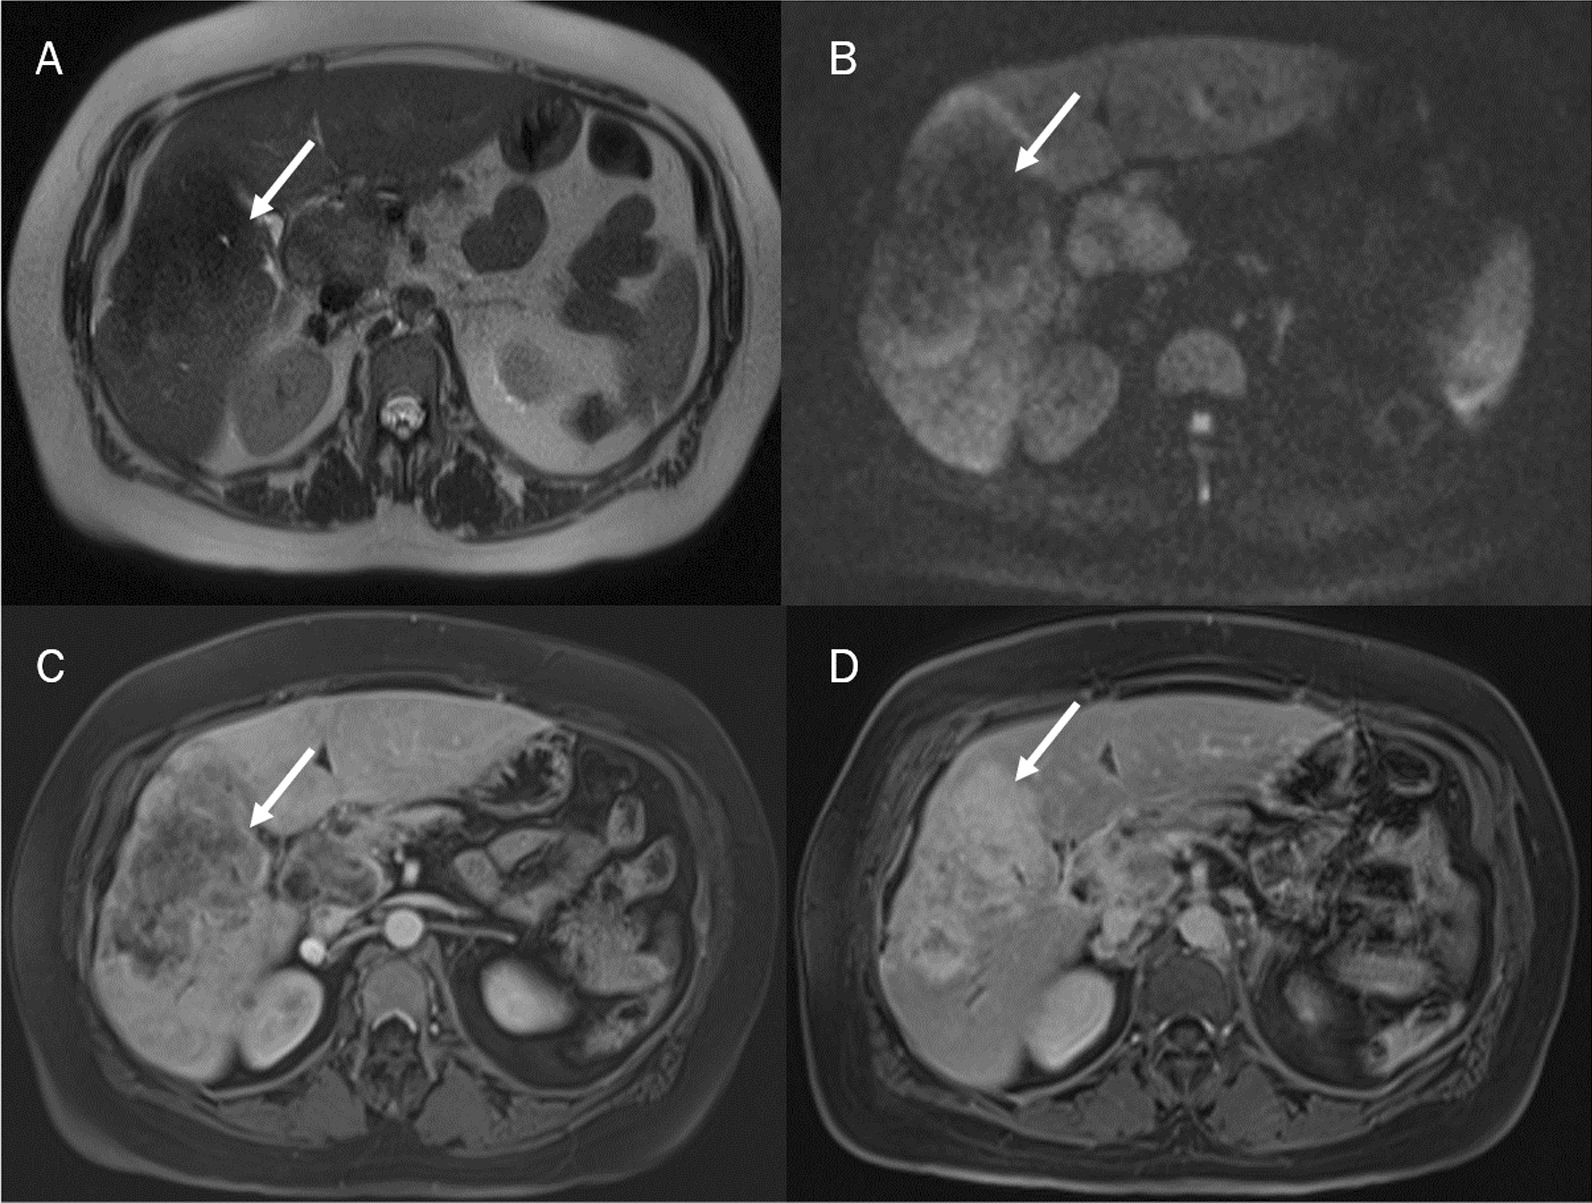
The targetoid appearance (TA) due to the fibrous stroma in the center of the ICC appears as a central area of less intensity, compared to a more hyperintense peripheral area on T2-W sequences (A) and DWI (B). The presence of this central fibrous stroma also characterizes the behaviour during the dynamic study. On dynamic contrast study, the mass-forming ICCS showed prominent peripheral rim enhancement (C: arterial phase) with centripetal or gradual progressive enhancement (D: delayed phase)
